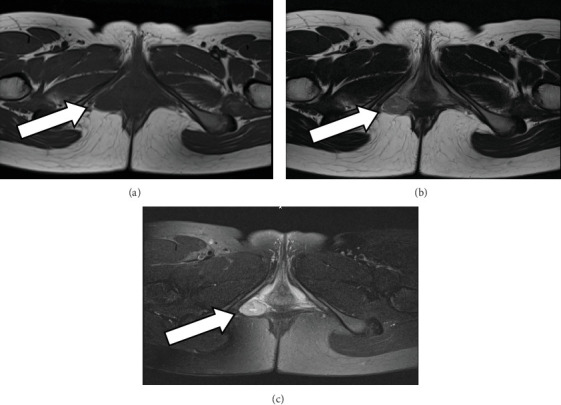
Imaging findings. Magnetic resonance imaging revealed a mass extending between the muscles of the transverse perineal muscle, the sciaticus spongiosus muscle, and the saphenofemoral muscle. (a) T1-weighted images and (b) T2-weighted images displayed low signal intensity, while (c) fat-suppressed T2-weighted images exhibited high signal intensity, indicative of a mesenchymal tumor.
